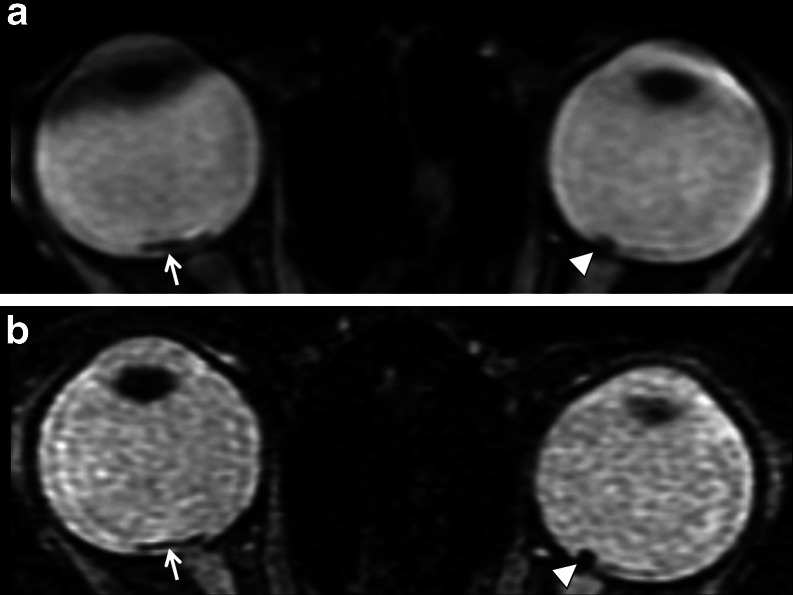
MRI on patient 6. Areas of low signal intensity consistent with hemorrhages are noted on SWI images on a 1.5T magnet on the standard brain MRI-SWI protocol (a) and on the dedicated high-resolution orbits SWI protocol (b). The hemorrhages are better delineated on high-resolution images (b). Other RH was also identified (not shown)
